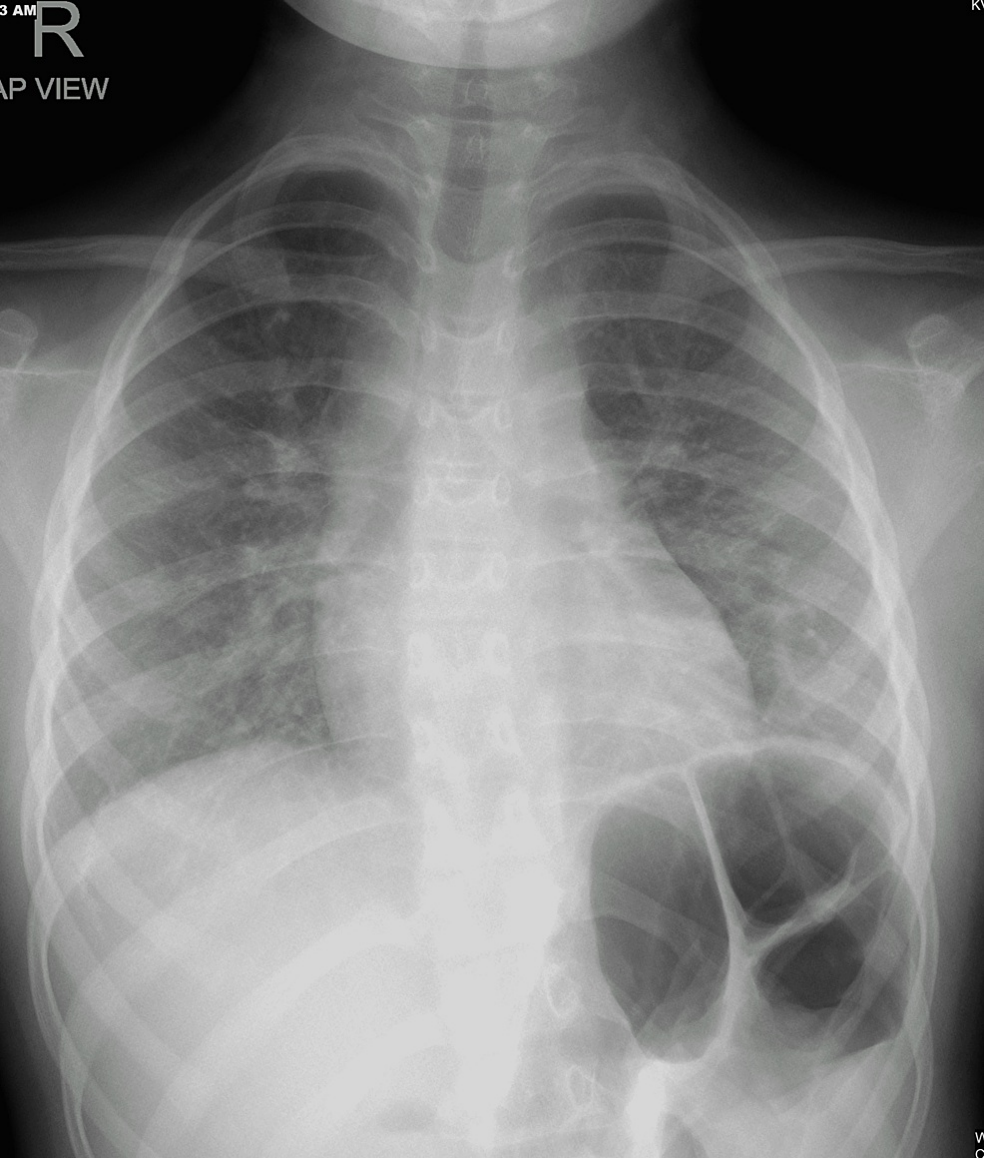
A follow-up chest X-ray showed significant improvement compared to the previous one.
